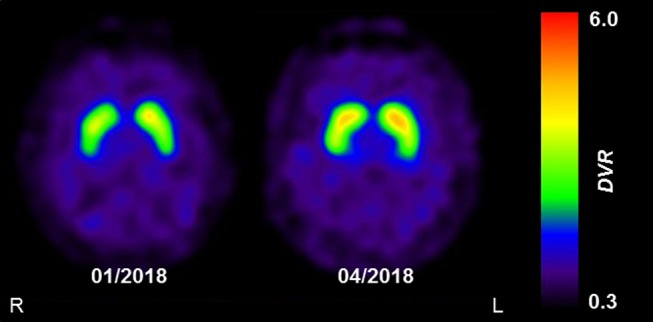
The effect of reserpine withdrawal on striatal DAT availability. Parametric images of striatal dopamine transporter (DAT) availability acquired using DAT-SPECT with [^123^I]FP-CIT. Images show the distribution volume ratio (DVR), which was used as a measure of DAT availability. The mild and homogenous decrease in DAT availability at initial presentation (01/2018) was completely resolved at follow-up (04/2018), 3 months after the withdrawal of reserpine. Images were acquired on a dual-headed SPECT system, 3 h after intravenous injection of 185 MBq [^123^I]FP-CIT.
